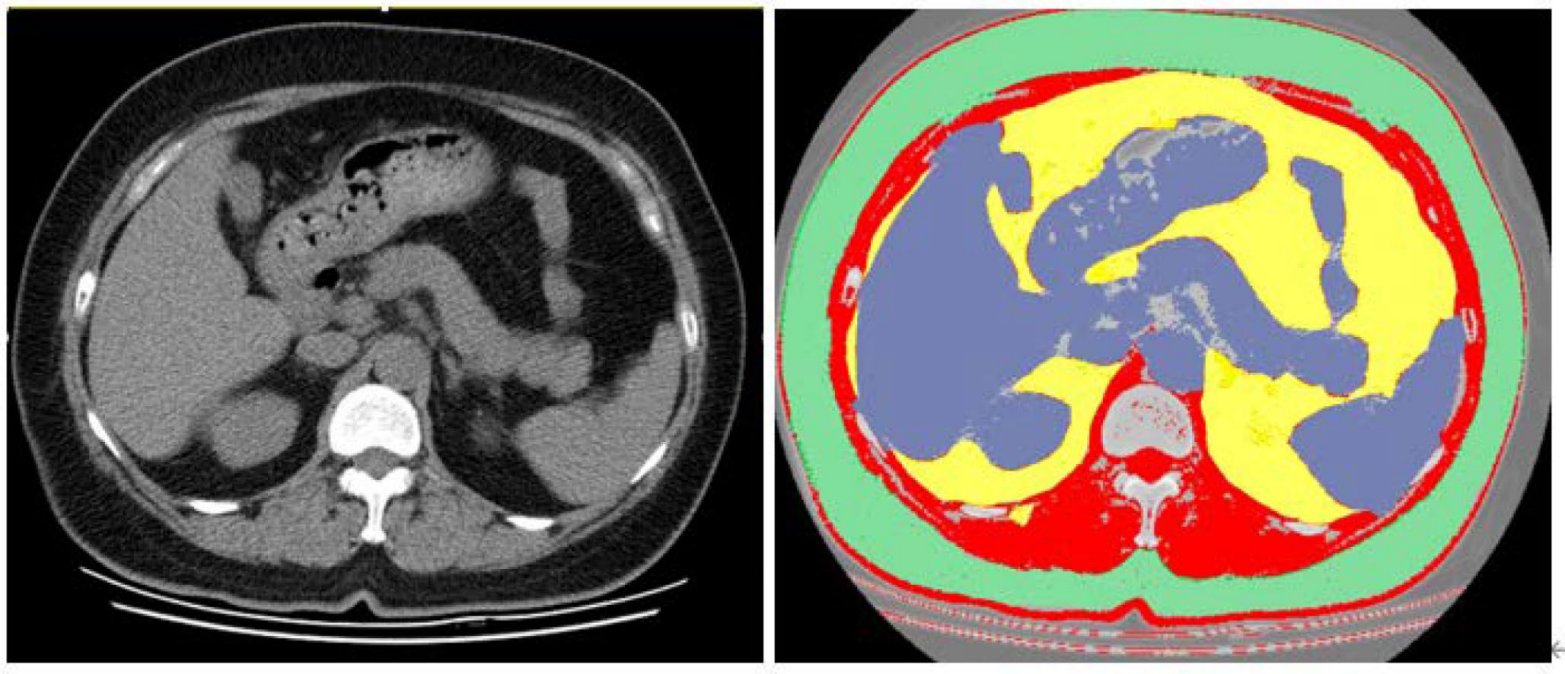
Representative segmentation of skeletal muscle area (red), visceral adipose tissue (yellow), and subcutaneous adipose tissue (green) from an axial image at the level of T12 of a CT scan. The HU values in the red part are − 29 to 150. The colored parts are quantified as areas (cm2) by the software. The skeletal muscle index was calculated as red area (cm²)/ height (m)²
